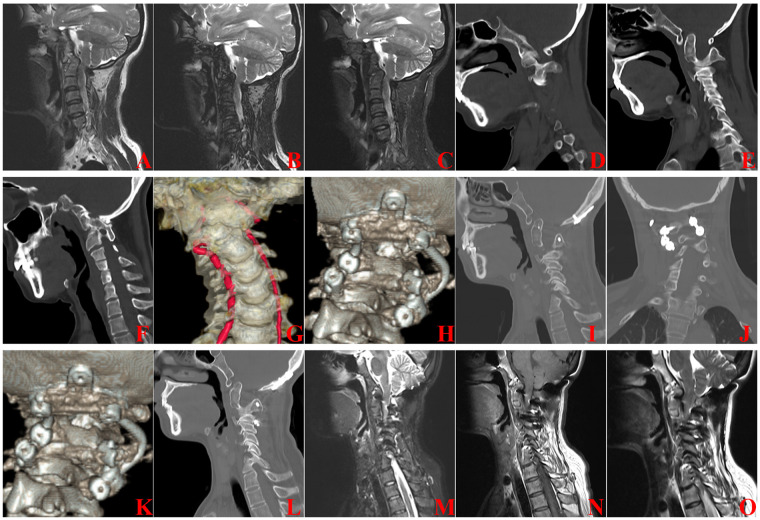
A 44-year-old female: (A–C) preoperative sagittal MRI revealed atlantoaxial dislocation with marked spinal cord compression; (C–G) preoperative CT demonstrated basilar invagination, occipito-cervical fusion, atlantoaxial dislocation, C2–3 vertebral block, and a high-riding vertebral artery; (H–J) CT at 1 year post-op confirmed satisfactory reduction of the atlantoaxial dislocation; (K–O) CT and MRI at 1.5 years both showed persistent decompression of the spinal cord.
